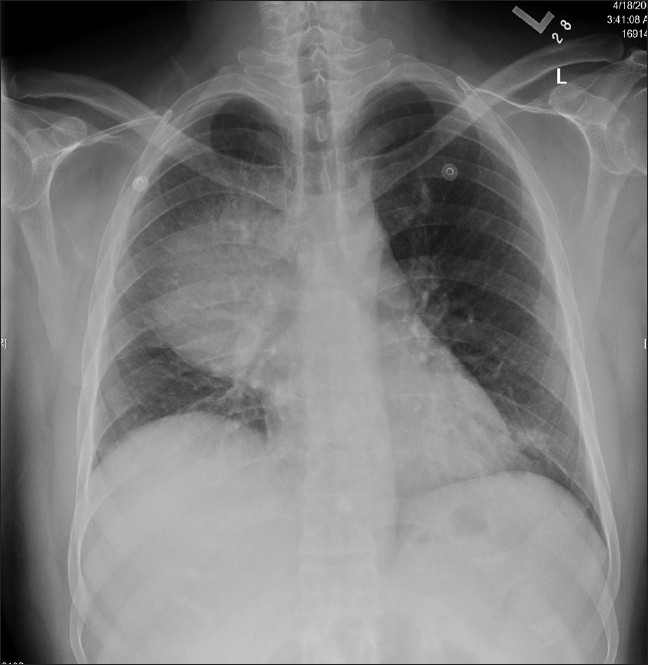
Admission chest X-ray showing large consolidation involving the right upper lobe
